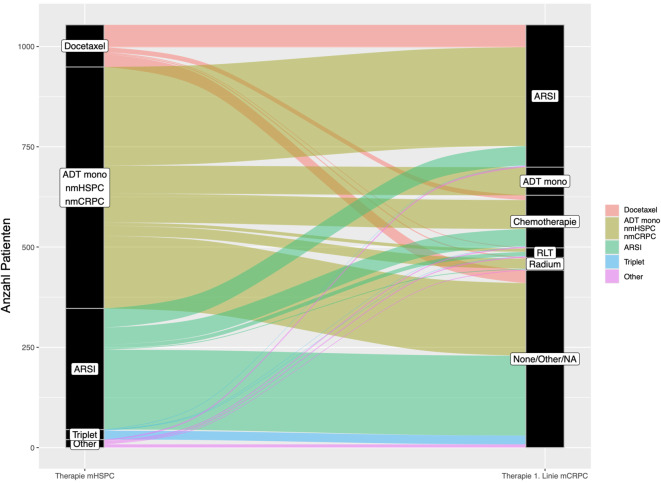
Sankey-plot-Darstellung der Therapielinien beim metastasierten hormonsensitiven Prostatakarzinom (mHSPC) und des metastasierten kastrationsresistenten Prostatakarzinoms (mCRPC). (*ADT* Androgendeprivationstherapie, *ARSI* Substanzen zur Blockade des Androgenrezeptorsignalweges, *RLT* Radioligandentherapie (Lutetium-PSMA), *nmHSPC/nmCRPC* nicht-metastasiertes mHSPC/mCRPC, *NA* unbekannt)
